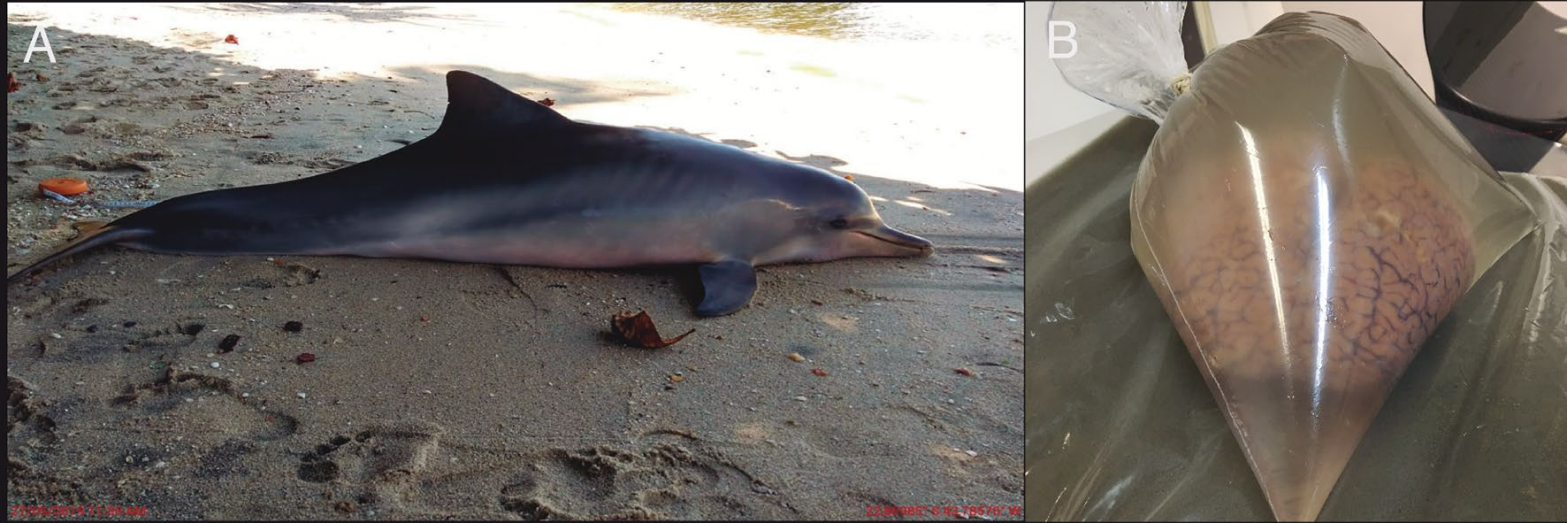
a Stranded adult female Guiana dolphin from Baía de Sepetiba, Rio de Janeiro, Brazil. The body length is 174 cm, b Guiana dolphin brain in a plastic bag filled with sodium azide phosphate buffer prior to MRI scanning
